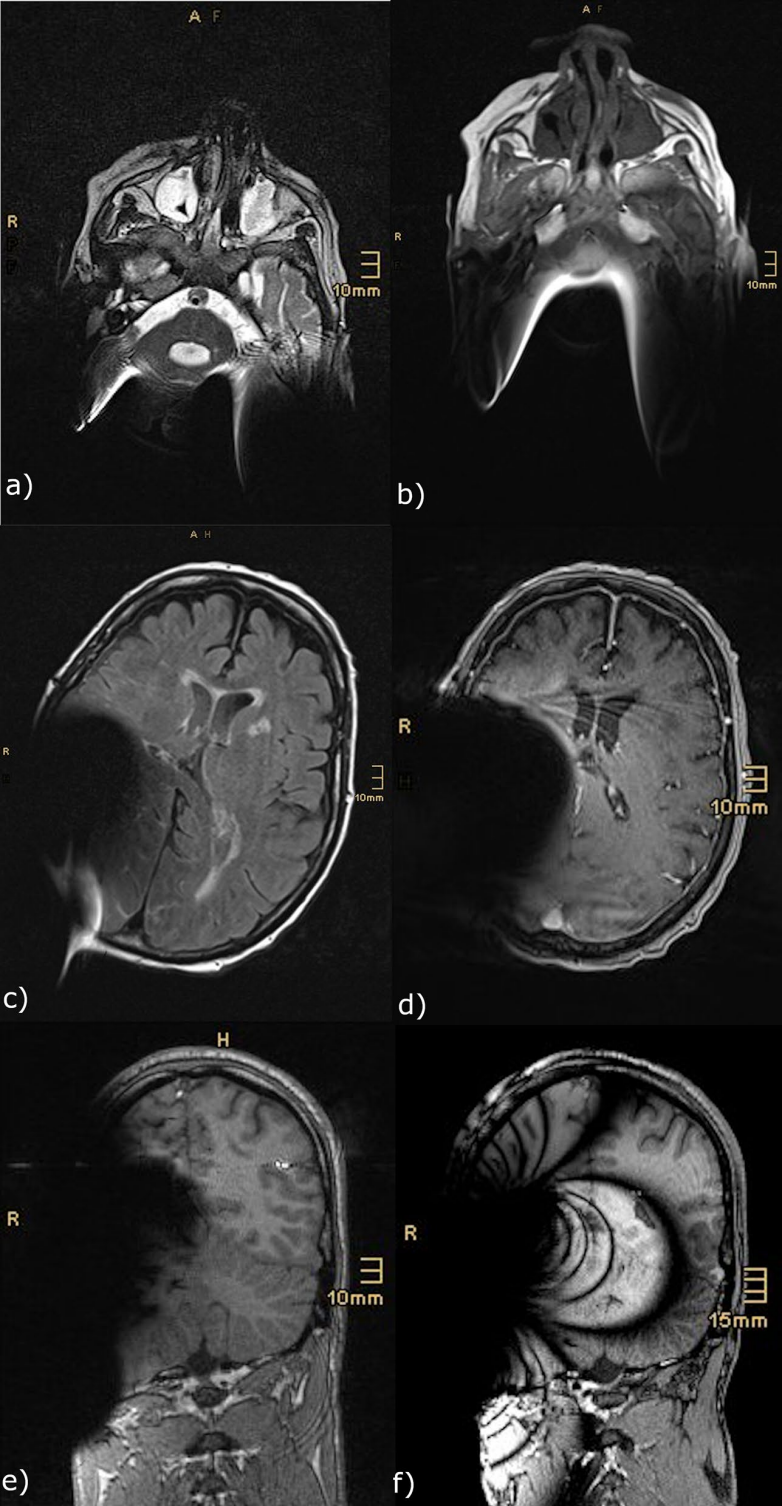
Examples of the retrospective data used in this study. The pictures (a, c) present different layers of 1.5 T T2 TSE sequences done in a patient with a cochlear implant on (a) both sides and (c) the right side. Pictures (b) and (d) show a comparable layer of the same patient done in a T1-mp_rage sequence. Picture e) visualize exemplary an artefact caused by a CI on the right side in a 1.5 T MRI (T1-mp_rage), while picture (f) represents nearly the same layer of an image done in a 3 T device.
